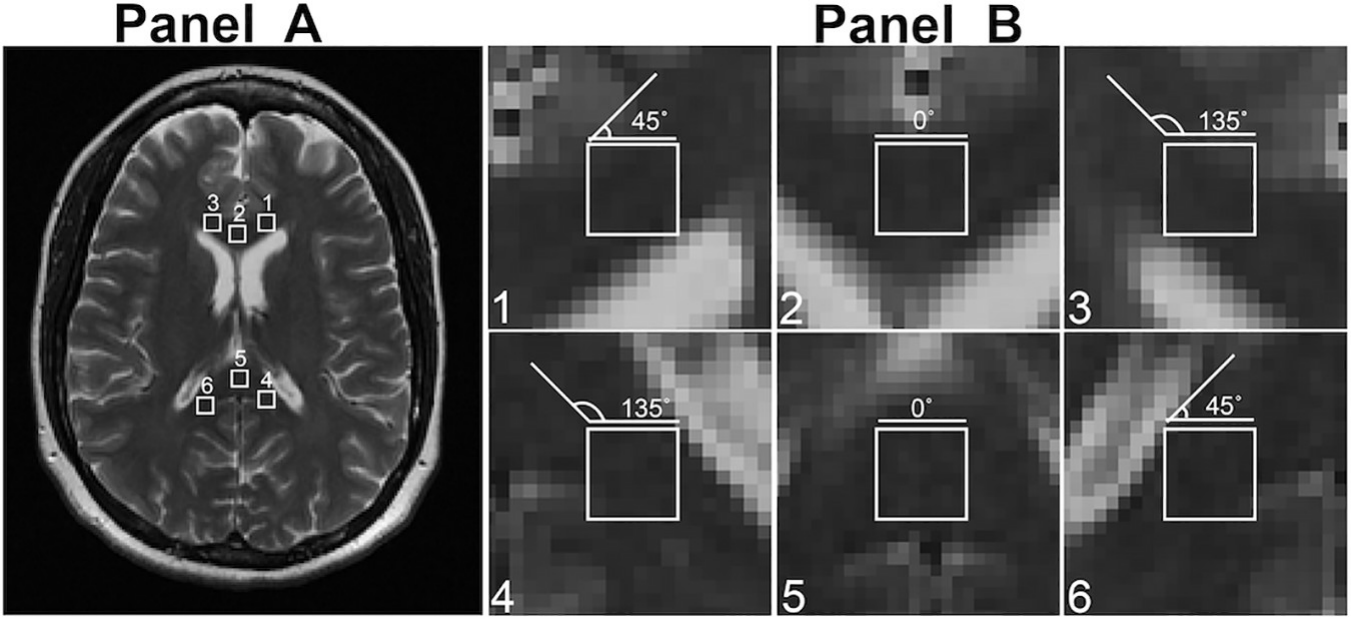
Representative ROIs along with predicted major aligning orientations based on the anatomy of corpus callosum. Panel A shows an example T_2_-weighted MR image from a control subject, where 6 ROIs are highlighted, located respectively in the left (1), central (2), and right (3) aspects of the genu and splenium (4, 5, 6). The predicted major orientations of these ROIs are: ROI 1 = ROI 6 = 45°; ROI 2 = ROI 5 = 0°; and ROI 3 = ROI 4 = 135°, similar to the trajectory direction of specific fiber tracks. Note that the same angle between paired ROIs (1 versus 6; 2 versus 5; 3 versus 4) reflect a near parallel trajectory direction of fiber tracks going through correspondent ROIs.
